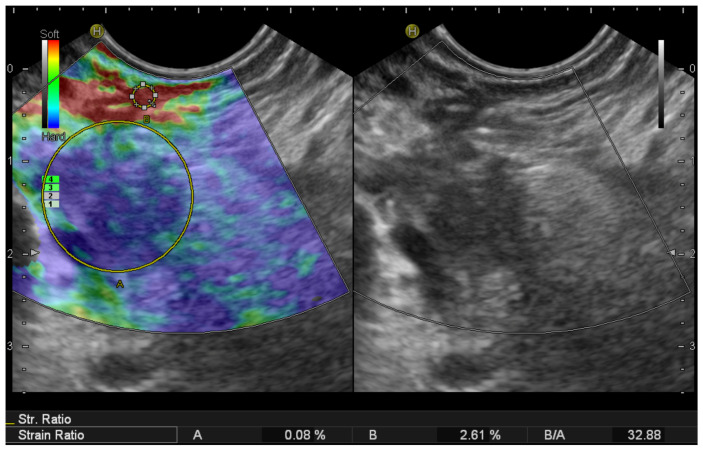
Endoscopic ultrasound elastography of a small pancreatic head neuroendocrine tumor. Two different areas (A, B) from the region of interest were selected for quantitative elastographic analysis. Area (A) is a lesion representative area including the biggest possible area of the tumor. Area (B) refers to soft (red) reference tissue outside the tumor. The quotient (B/A) (strain ratio) is considered as the measure of the elastographic evaluation.
